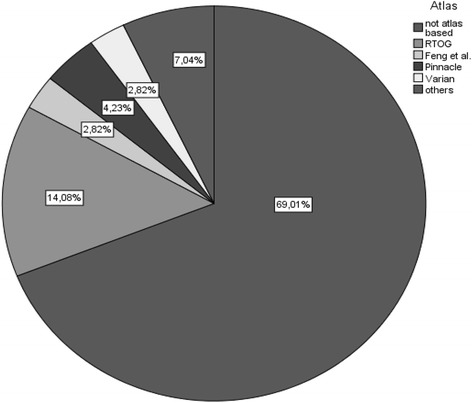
Atlas based contouring of the heart during routine treatment planning. “others” included several different diagnostic CT atlases available in the departments
